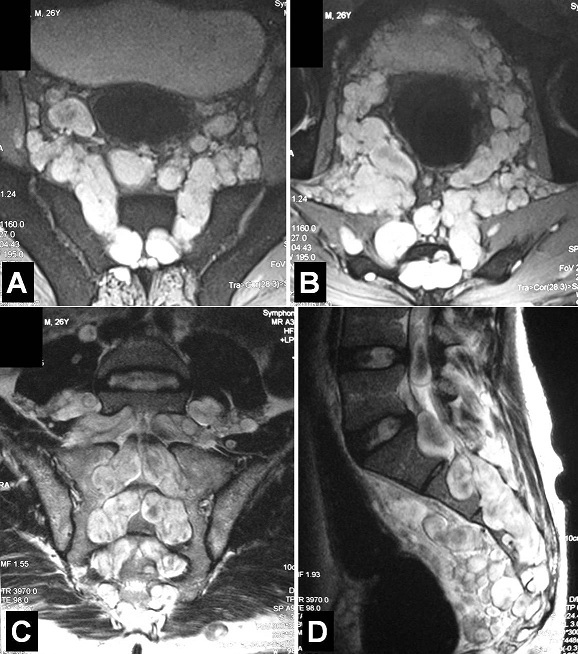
T2-weighted MRI of the lumbosacral area on axial (A and B), coronal (C) and sagittal (D) views. Multiple intracanalar hyperintense lesions of the lumbosacral spine causing scalloping of posterior parts of all the sacral vertebrae and neural foraminal widening with bilateral and symmetric extension (dumbbell formation) to the pelvic region
